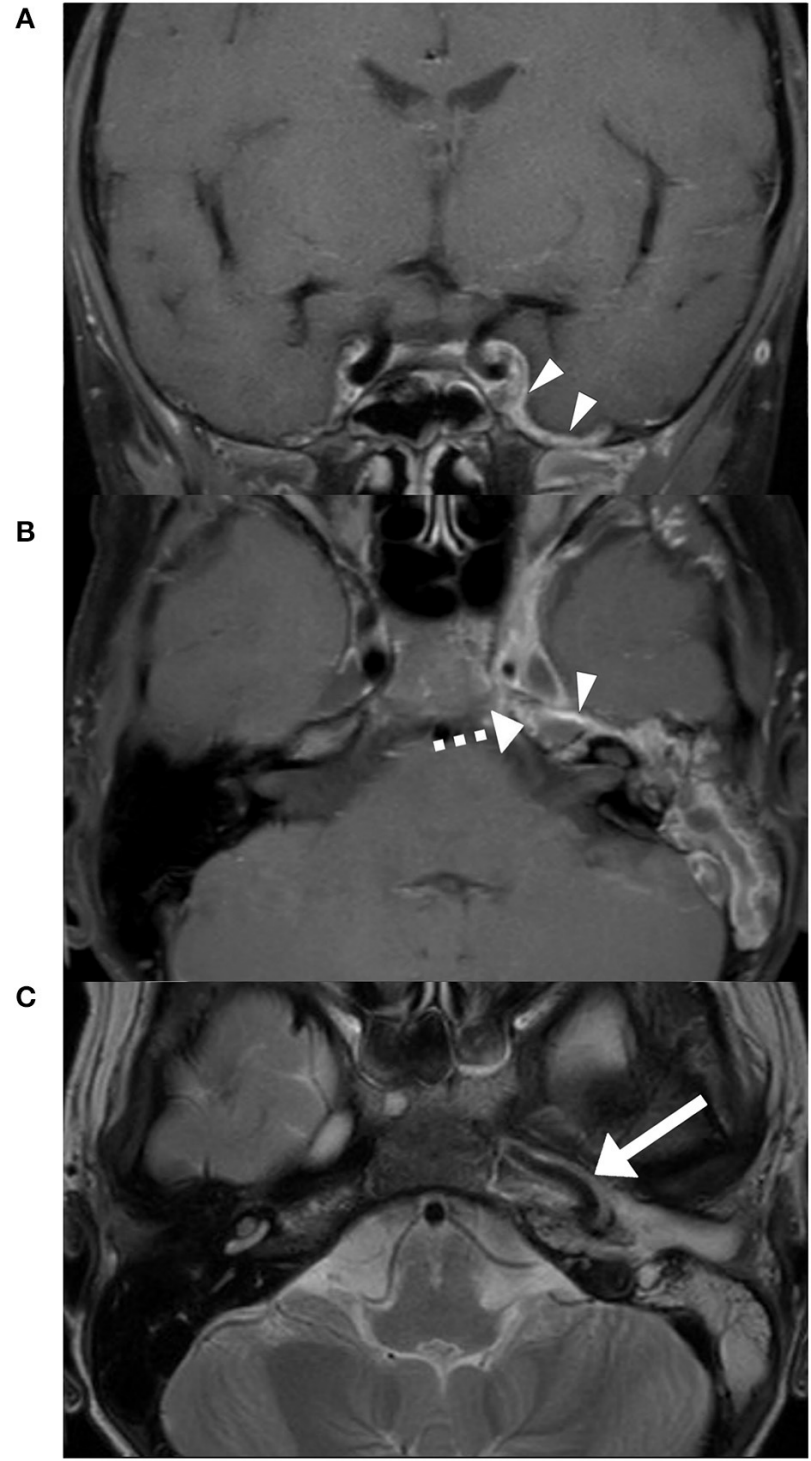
Initial MRI T1 DIXON FS post gadolinium. Dural enhancement (triangles) in the area of the left temporal lobe (A). Anteromedial rim enhancement of the opacified petrous apex (dashed arrow) (B). Thrombotic cavernous sinus and a marked vasculitis of the internal carotid artery (arrow) on the left side (C).
